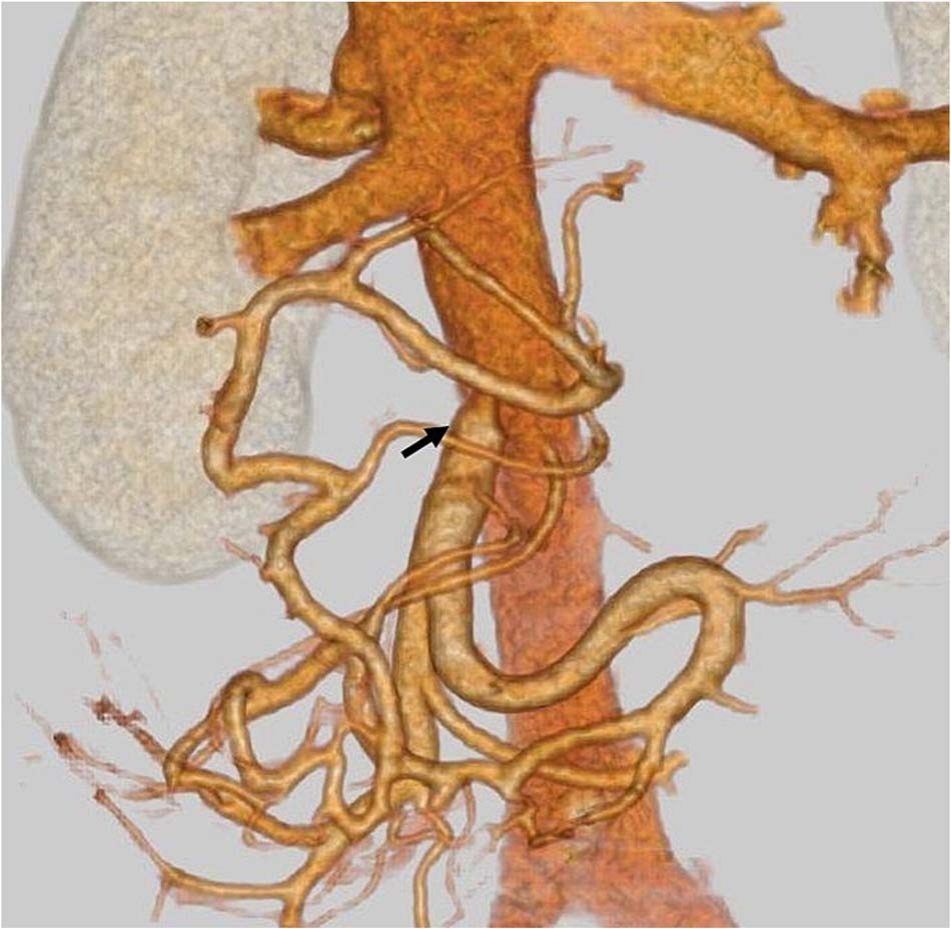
Postoperative contrast-enhanced CT: An arrow indicates patent mesocaval shunt (on postoperative day 14).
